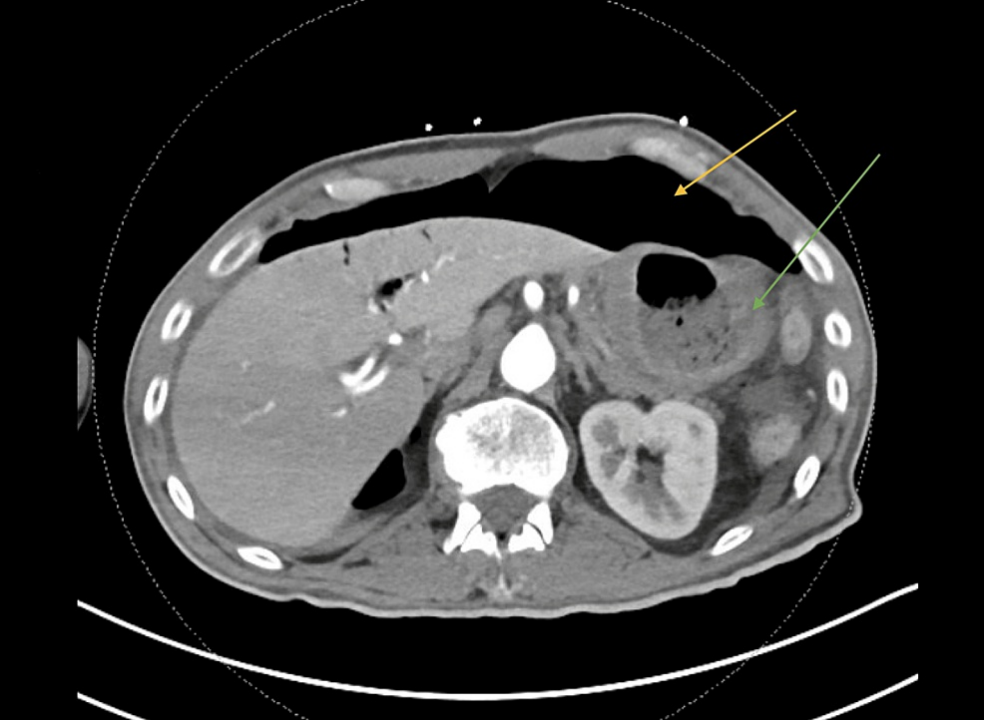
CT scan of the abdomen with intravenous contrast showing large-volume peritoneum Large-volume peritoneum is shown by yellow arrow and stomach wall thickening is shown by green arrow. CT, computed tomography.
